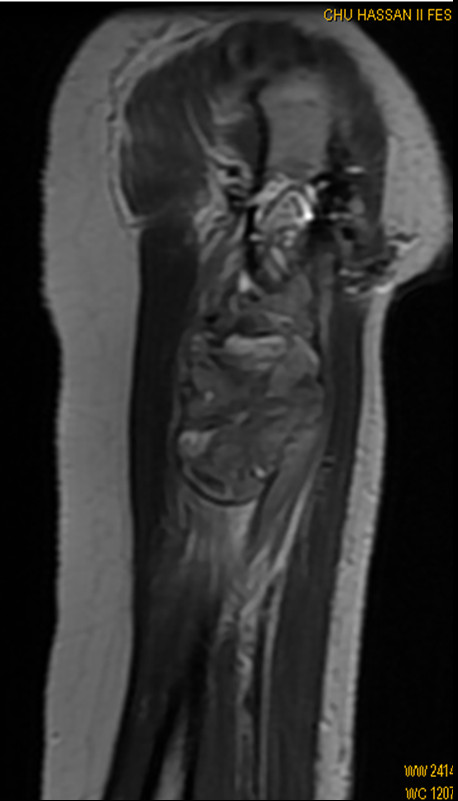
Sagittal T1 - weighted MR image at the time of first presentation, reveals a huge intra- and extraosseous tumor of the proximal humeral diaphysis.
